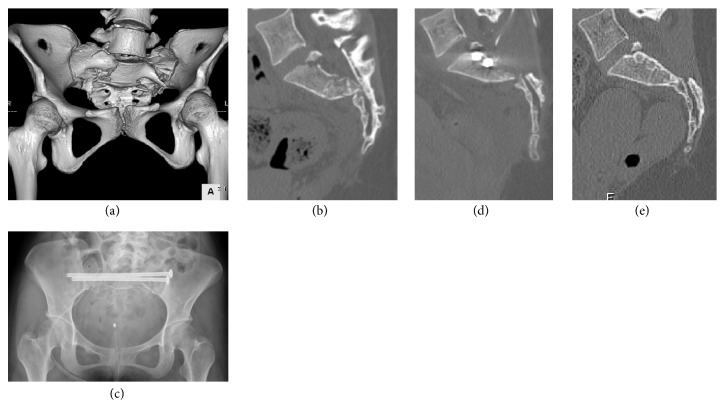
Case 9. An 18-year-old man who was injured from a fall from a height underwent S1 and S2 TSTI full threaded screw fixation for a Roy-Camille type 2 U-shaped transverse sacral fracture. (a) Preoperative 3-dimensional CT reconstruction of the pelvis is shown. (b) Preoperative CT scan of the sacrum in the sagittal plane is shown (preoperative sacral kyphotic angle: 89°; fracture translation: 8 mm). (c) Postoperative anteroposterior radiograph of the pelvis is shown. (d) Postoperative CT scan of the sacrum in the sagittal plane is shown (preoperative sacral kyphotic angle: 32°; fracture translation: 3 mm). (e) Final follow-up CT scan of the sacrum in the sagittal plane is shown. Good reduction quality was maintained.
